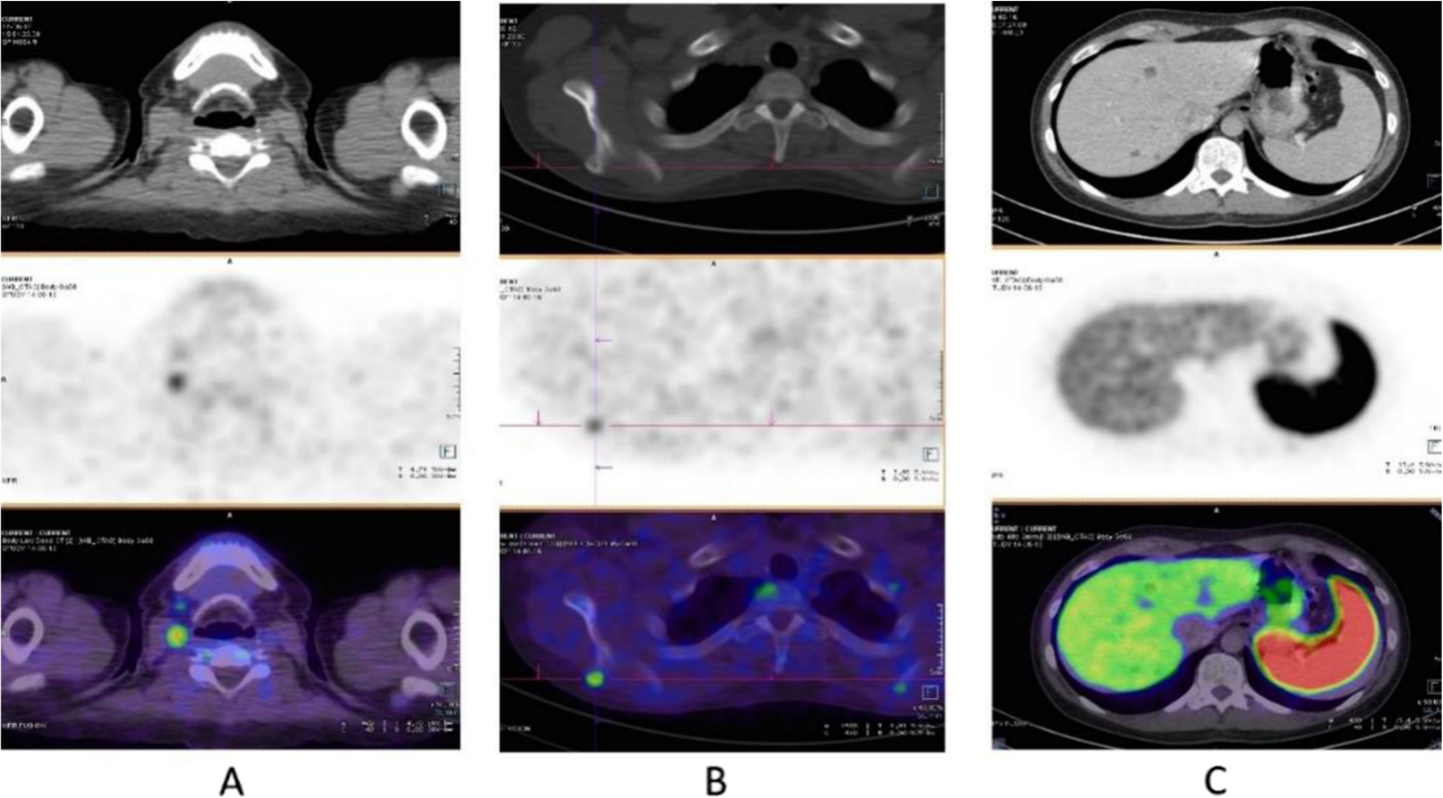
^68^Ga-DOTA peptide, recurrent medullary thyroid cancer (MTC). *Clinical history*: 56 y.o. man with previous history of MTC, treated by surgery. Progressive increase in calcitonin (TCT = 19200). *PET/CT findings*: increased uptake in cervical LN (a) and bone lesion (b right scapula). Additional hepatic metastases are seen on the diagnostic CT, but due to the high background they are not evident on PET images (c)
